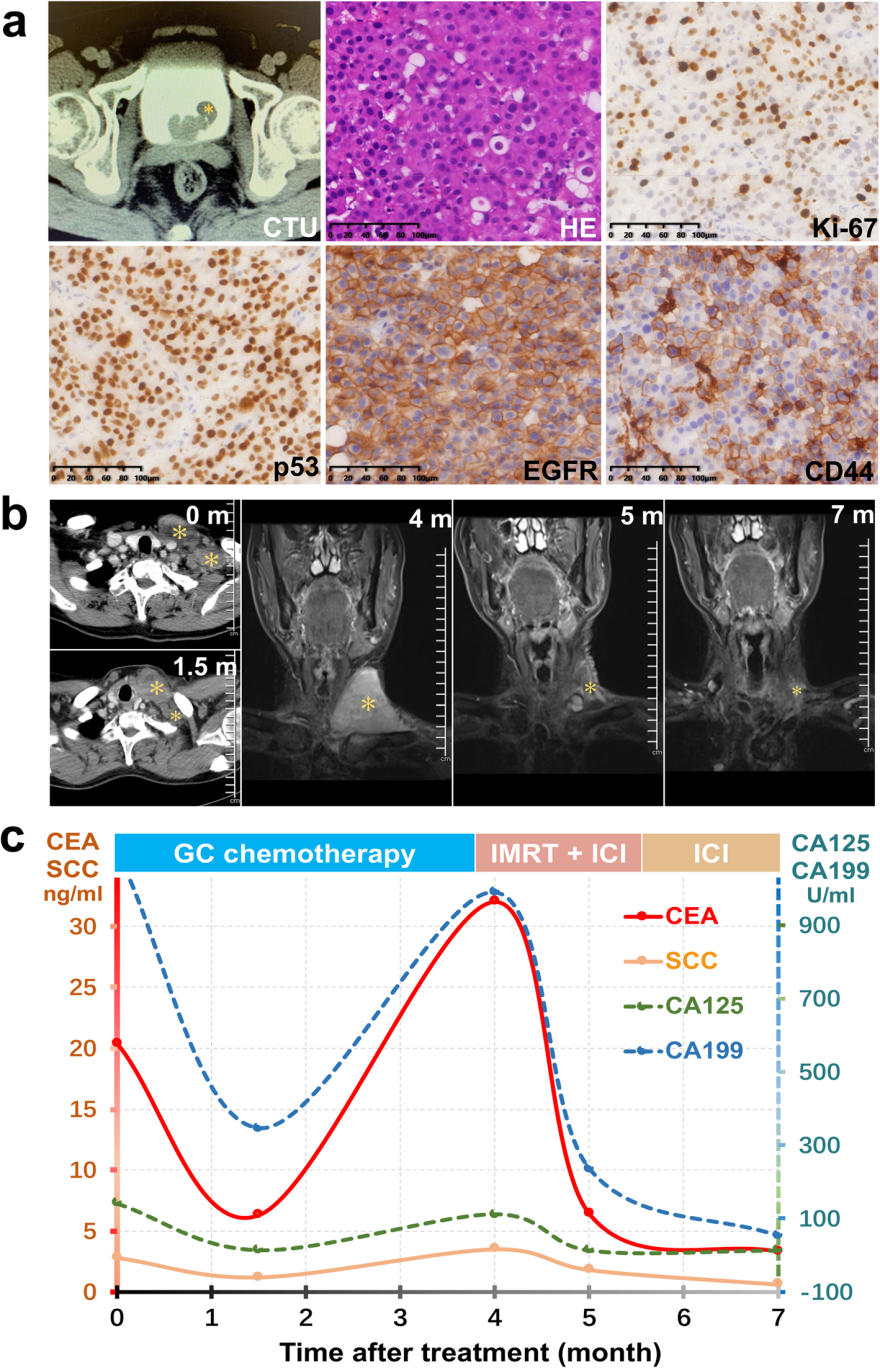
Presentation of the mNMIBC case from our center: CT urography and pathologic findings of primary tumor (a); dynamic changes of cervical lesion in CT/MR scans (b) and fluctuating levels of serum tumor markers (c) during post-metastatic treatment (yellow asterisk referring to primary or metastatic lesions)
